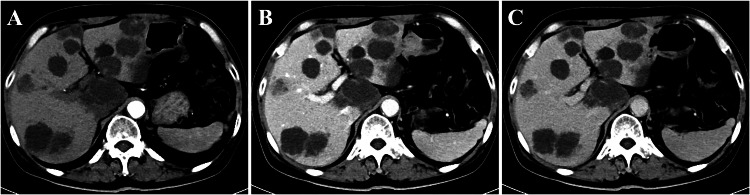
Abdominal contrast-enhanced CT in a 54-year-old female with liver metastases. A Early arterial phase (misclassified as late arterial phase by the two-step model). B Correctly identified portal venous phase. C Correctly identified delayed phase
